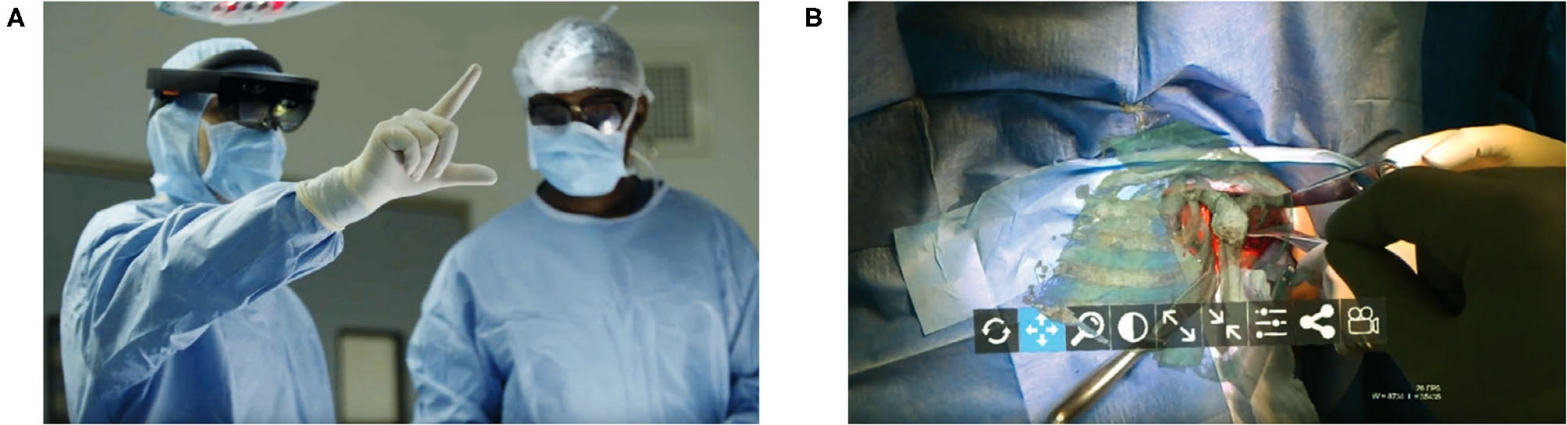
A proof of concept application of *Microsoft Hololens* for reverse total shoulder arthroplasty. (A) The surgeon is able to view in real-time and place in space a 3D hologram from a CT of the patient's scapula. (B) A 3D hologram of the patient's scapula is superimposed intraoperatively in order to fully visualize the glenoid and other relevant anatomy. Reproduced with permission by Gregory et al. (74).
